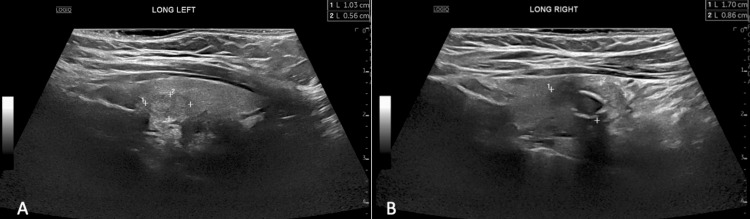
Thyroid gland ultrasound of left (a) and right (b) nodules, which pathology showed to be papillary thyroid carcinoma. A) Largest nodule in the left lobe measuring 1.2x1.0x0.9cm is hypoechoic with no associated calcifications. B) Largest right nodule measuring 1.7x0.8x0.9cm is hypoechoic with coarse peripheral calcifications.
